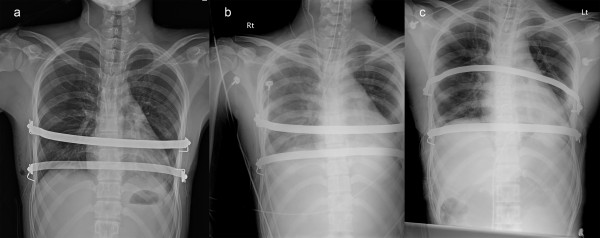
Serial chest x-ray after operation. (a) Chest x-ray at the next morning after Nuss procedure showing two bars and hemopneumothorax was not noted (b) 2 hours after rounding, right-side hemothorax was revealed. (c) After reoperation, hemothorax disappeared.
